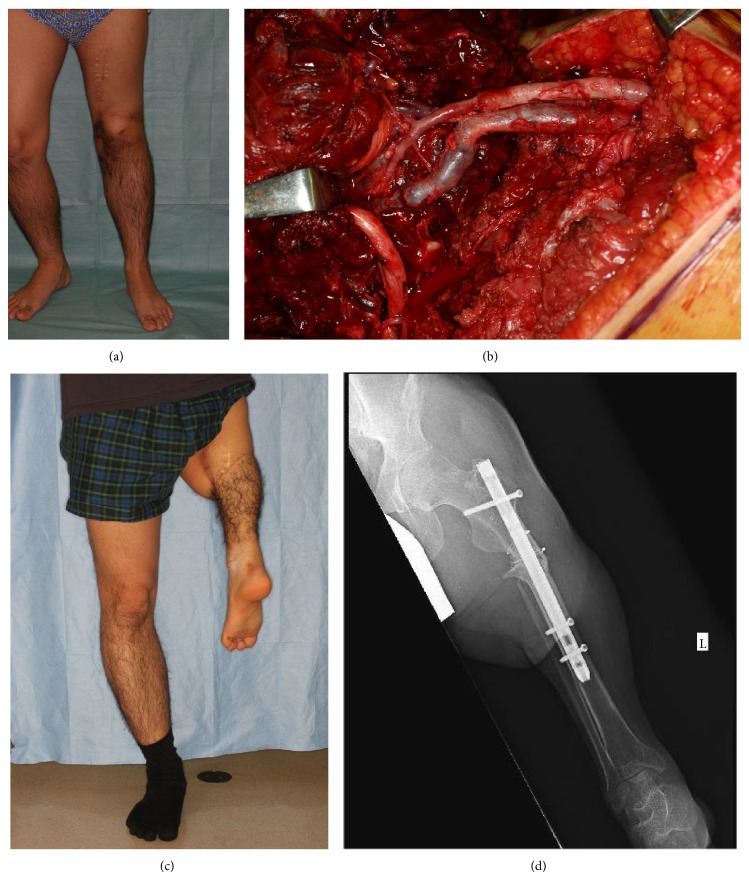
(a) Preoperative status with decreased range of motion due to infection of the prosthetic knee joint, (b) vascular reconstruction after rotation of the distal leg, (c) appearance 3-year after rotationplasty, (d) X-ray finding 3-year after rotationplasty.
